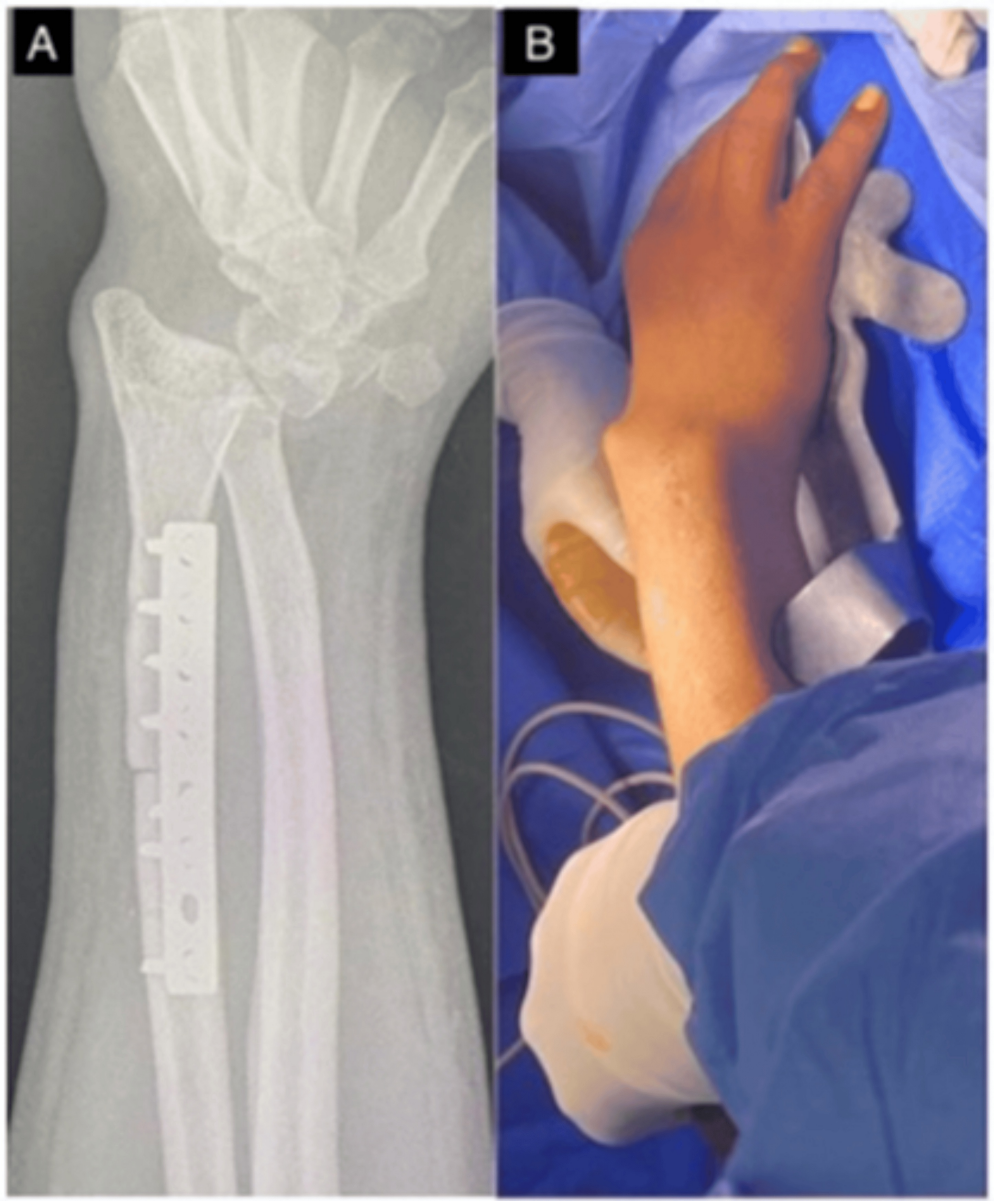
Lateral radiograph (A) and intraoperative clinical photograph (B) showing wrist subluxation.
